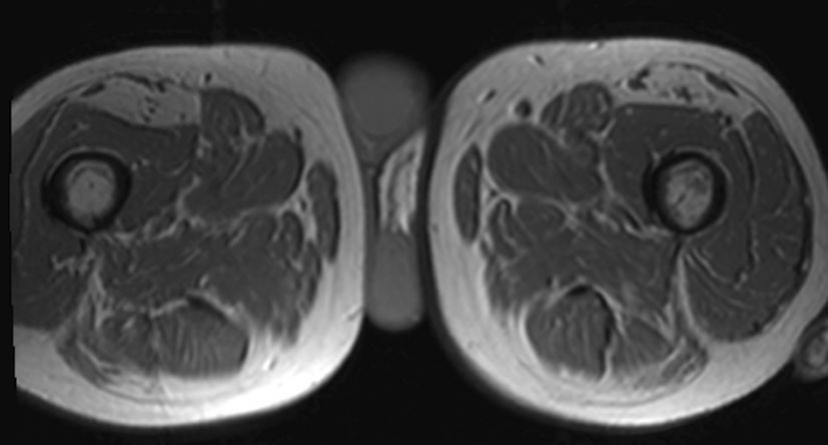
Vitamin D deficit in patients with selective atrophy of rectus femoris as shown by standard MRI of both thighs of an elderly man with 25-hydroxyvitamin D level of below 12 ng/mL
